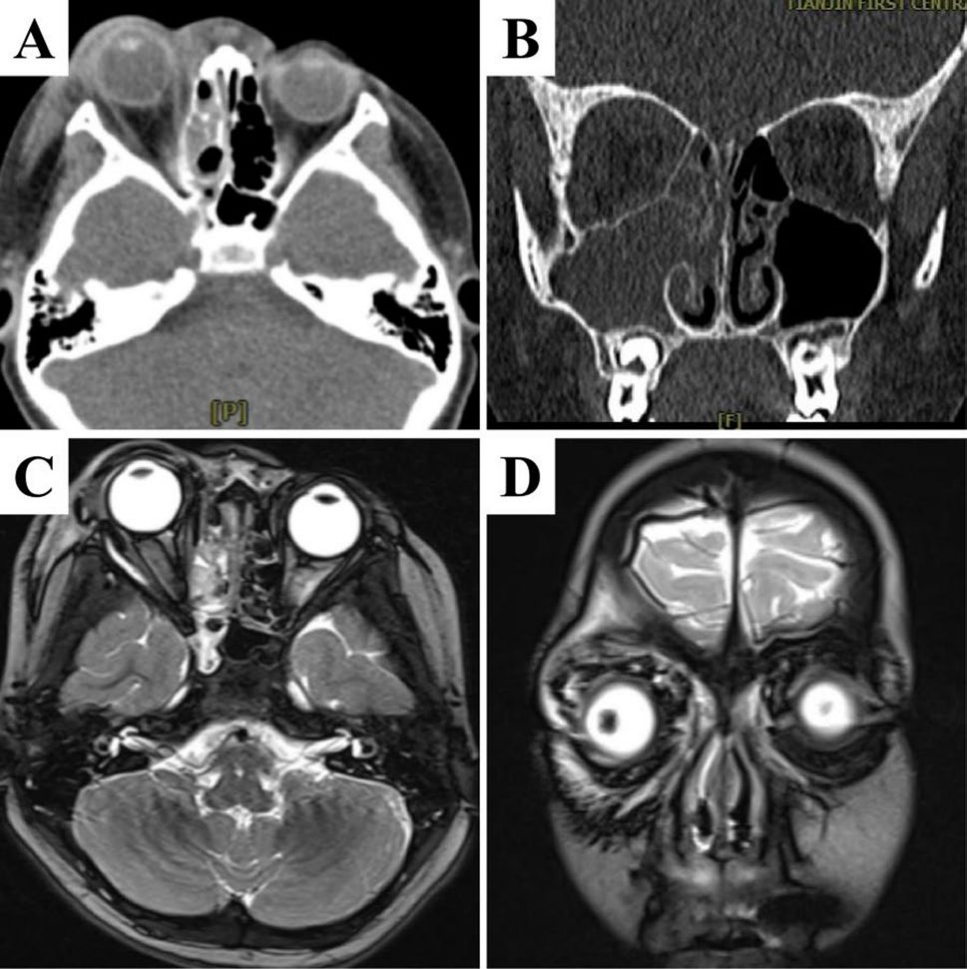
Imaging findings of the patient. a and b Preoperative orbital CT scans: Periorbital soft tissue swelling with pneumocele, findings consistent with orbital cellulitis. c and d Preoperative sinus MRI scans: Inflammation is present in the right maxillary sinus, ethmoid sinus, and sphenoid sinus, accompanied by a right orbital abscess
